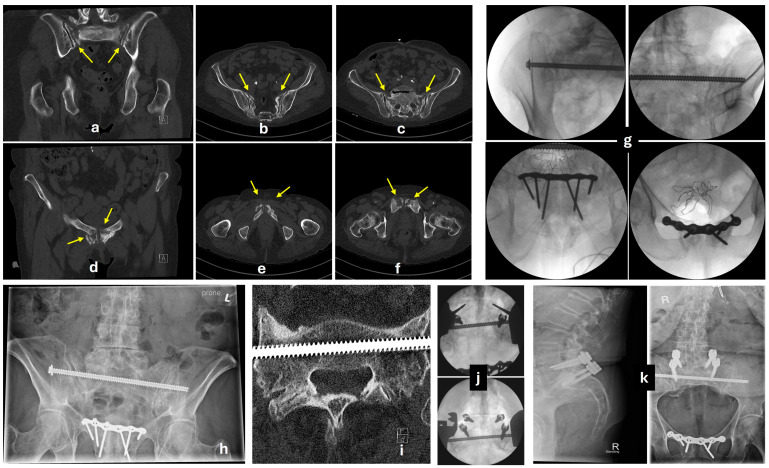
Case example of an insufficiency pelvic fracture. A 77-year-old female patient with severe osteoporosis, history of hysterectomy at a young age, bladder neck surgery, multiple fragility thoracolumbar fractures, was referred with worsening lower back and sacral pain for the last 5 months, without any history of trauma. MRI evidence of a high sacral H-type insufficiency fracture (transverse plane at S2). (a–c) CT-scan showing nonunion of the sacral fractures (yellow arrows), bilateral fractures of the L5 transverse processes, and (d–f) nonunion of the bilateral para-symphyseal fractures of the rami (yellow arrows). (g) Fluoroscopic views during the open reduction internal fixation of the rami fractures with a 3.5 mm reconstruction plate bridging the symphysis pubis and a single transiliac transsacral 8.0 mm position screw at S1 level. Full bone metabolic profiling was performed, and intravenous supplementation of her calcium and vitamin D levels was provided. She was allowed immediate mobilisation, weight bearing as pain permitted, with the use of a Zimmer frame. She gradually weaned off the frame and managed to mobilise with a single stick after the first two months. (h) Anteroposterior pelvic X-ray at 8 months. As expected, non-symptomatic loosening of the anterior fixation has occurred. At 12 months, she developed a new onset of severe back pain with additional neuropathic L5 nerve distribution. (i) CT evidence of complete union of the sacral fractures, no metalwork impingement. An MRI scan identified severe degeneration of the facet joints, bulging discs at L3/4 and L4/5 levels, stenosis of the right L5 neural foramen, and spondylolisthesis. (j) One year later, she underwent root decompression of right L5-S1 and L5-S1 fusion, with partial recovery of her neuropathic pain and improved mobility at the latest follow-up. (k) Anteroposterior and lateral X-rays at 12 months following the last procedure.
